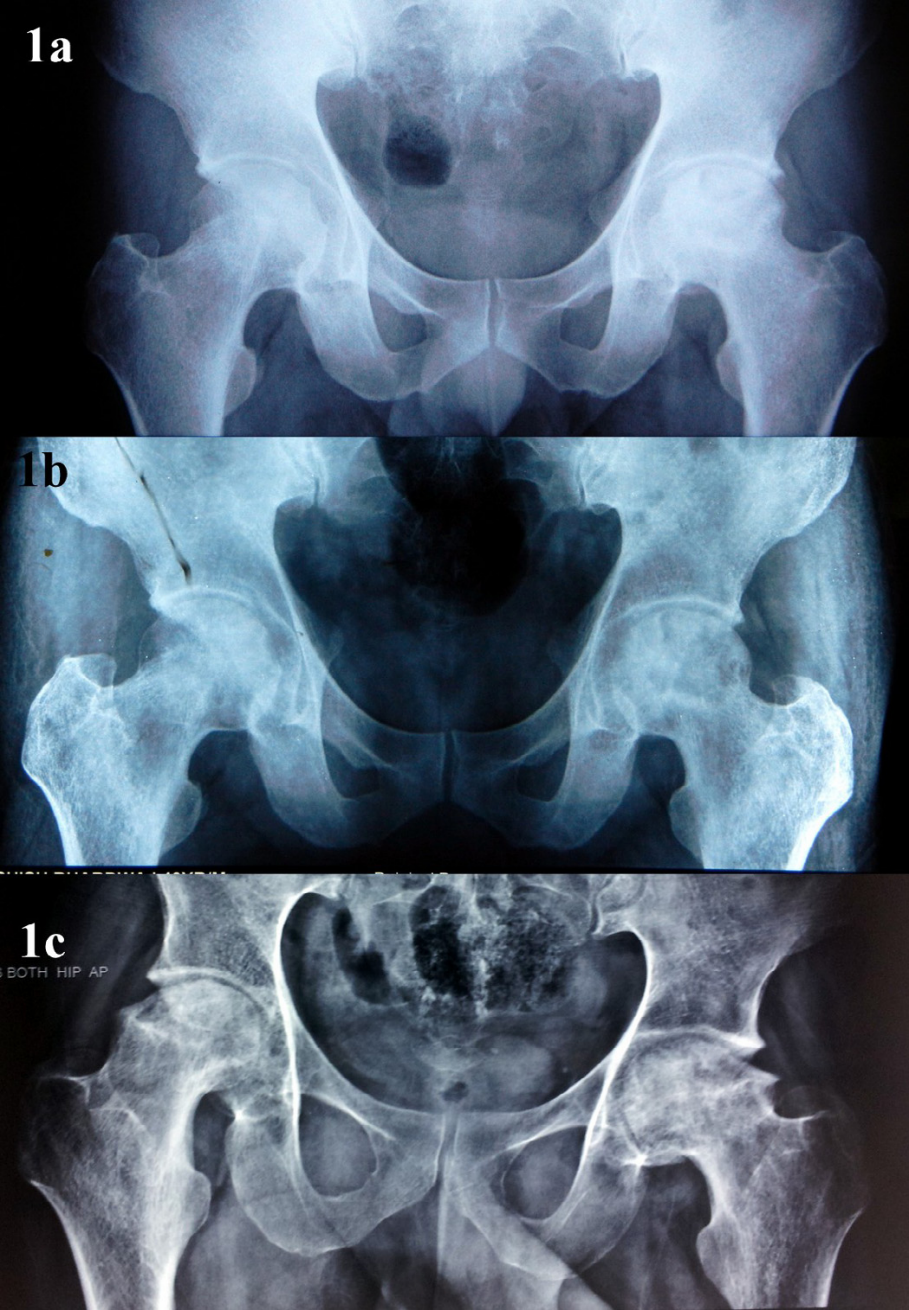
Serial radiographs of a 40 year old male with ONFH bilaterally: panel a: early stages showing density changes, more pronounced in left hip; panel b: after 3 months, fragmentation changes have appeared in both femoral heads. Femoral head collapse is eminent at this point; panel c: 1 year follow-up shows partial collapse in both femoral heads, relatively preserved joint space, relative flattening at the lateral periphery of the femoral heads, and formation of impinging cam-type lesion.
